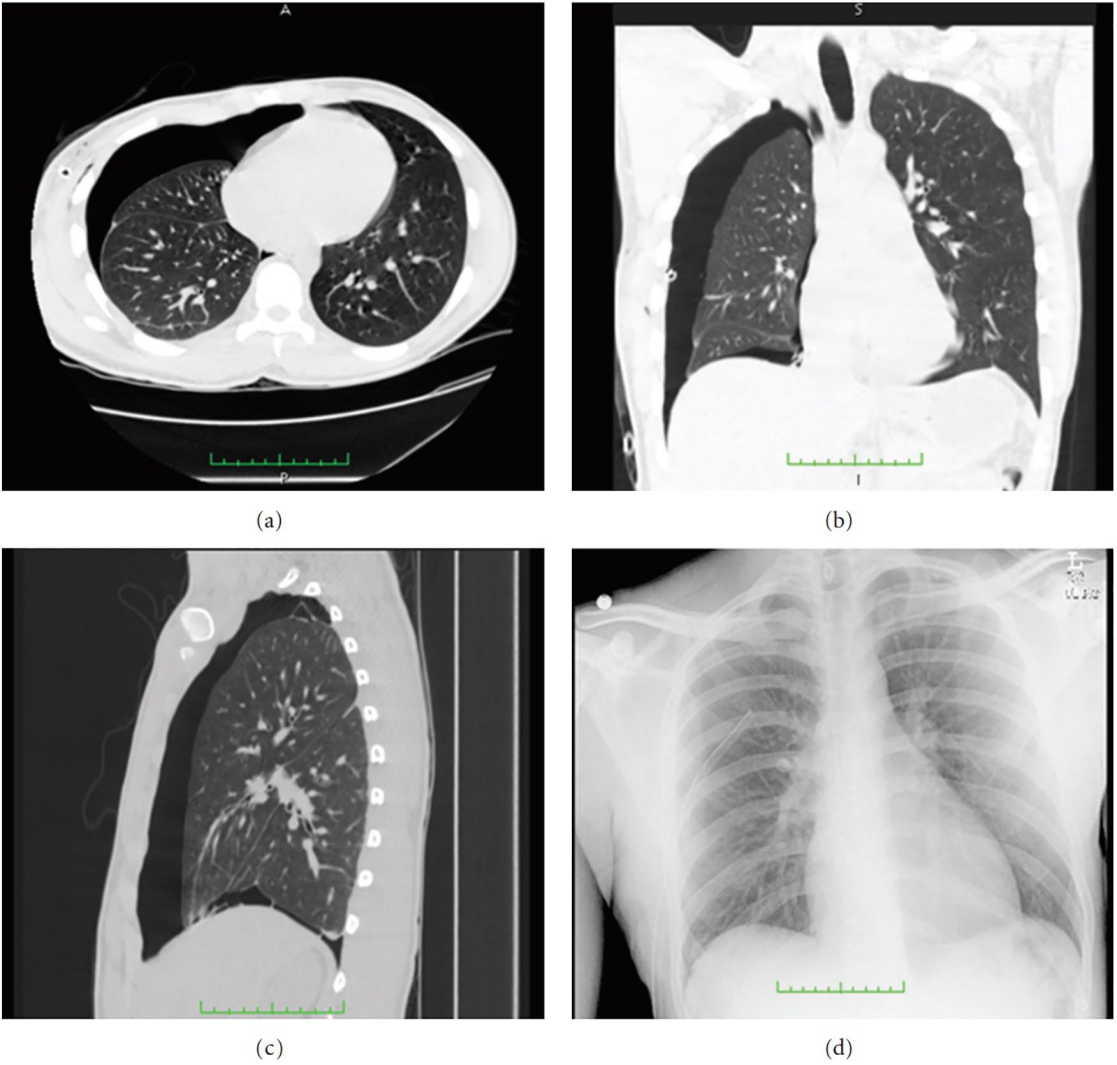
The CT images in transverse (a), coronal (b), and sagittal (c) views reveal a moderate to large pneumothorax in a patient, while the chest X-ray image (d) of the same patient taken two hours earlier lacks the level of detail provided by the CT images. The CT images are more effective in detecting and measuring the size of the pneumothorax.
